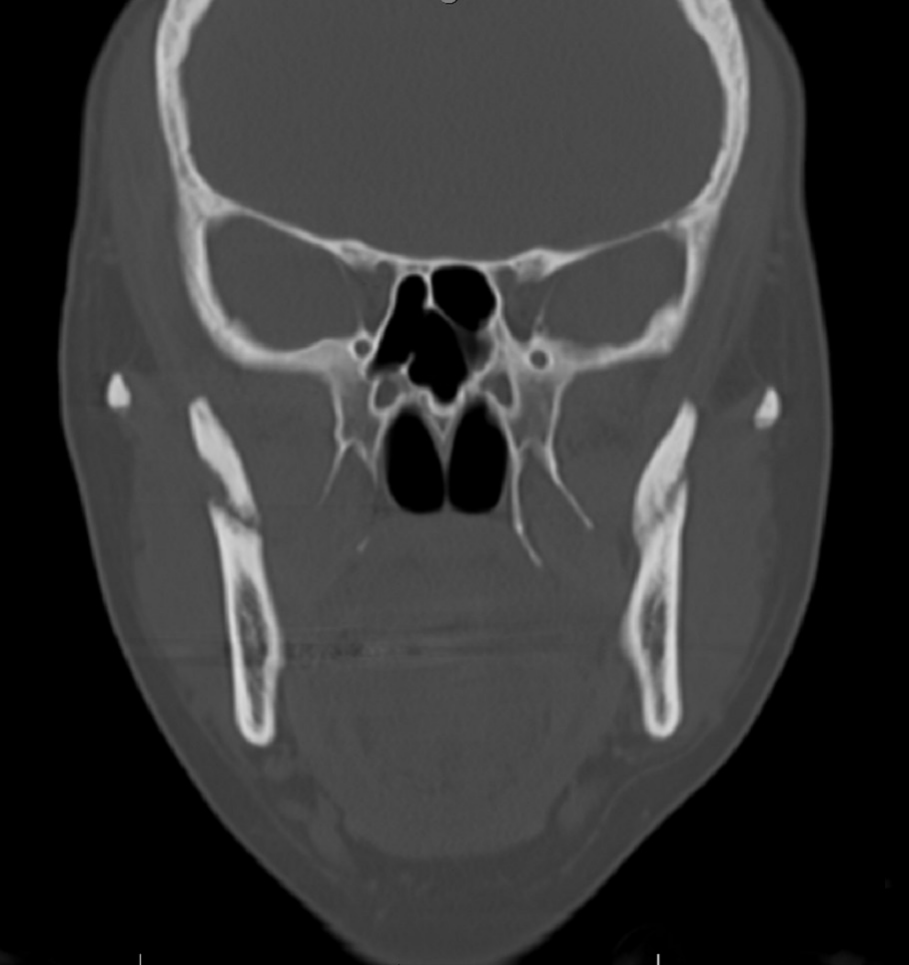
Coronal CT shows isolated bilateral coronoid process fracture
